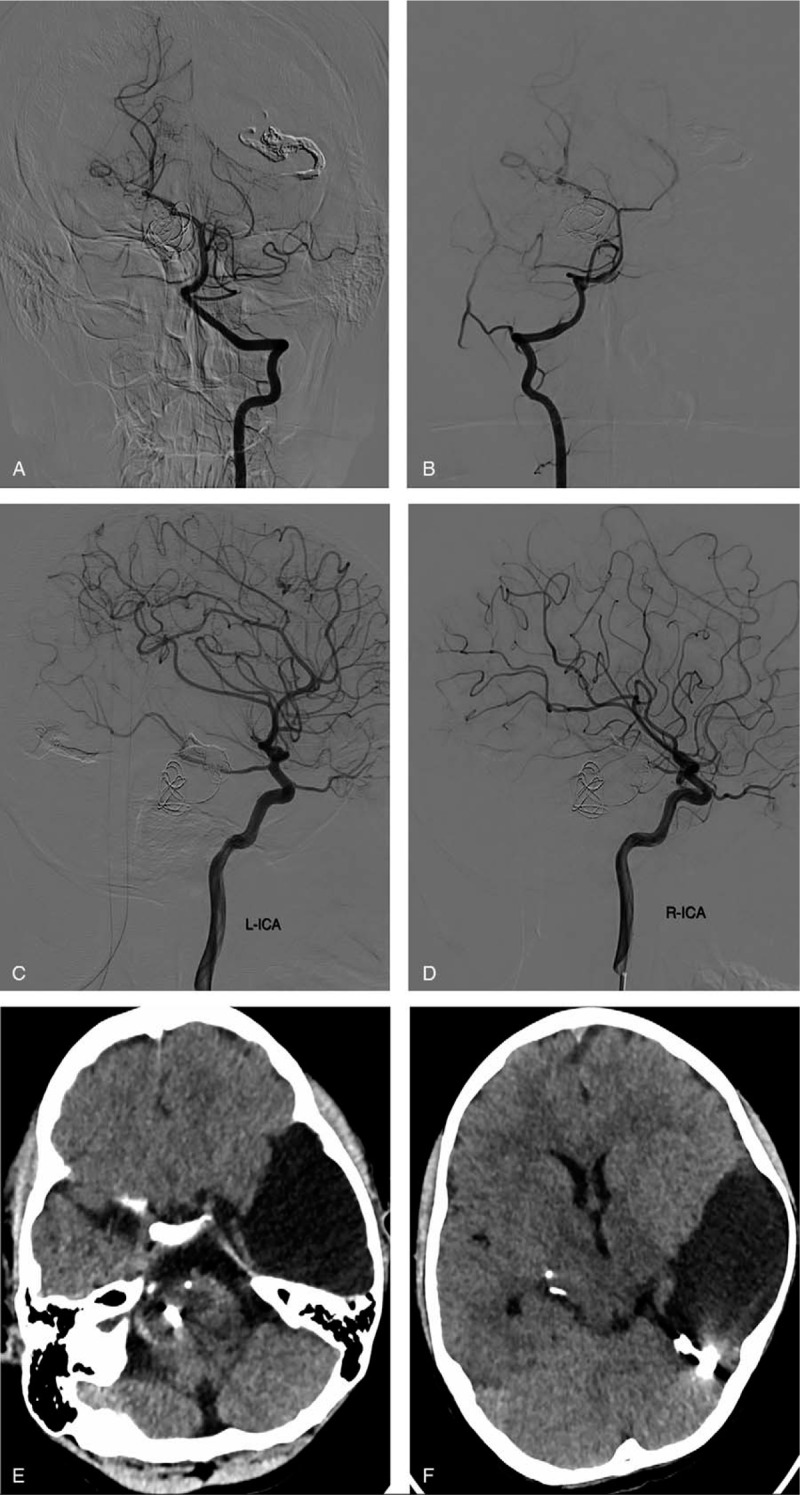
Six months follow-up images. (A–D) digital subtraction angiography images showing total disappearance of the biteral pial arteriovenous fistulas. (E, F) Computed tomography scan images showing no regression of the cyst.
